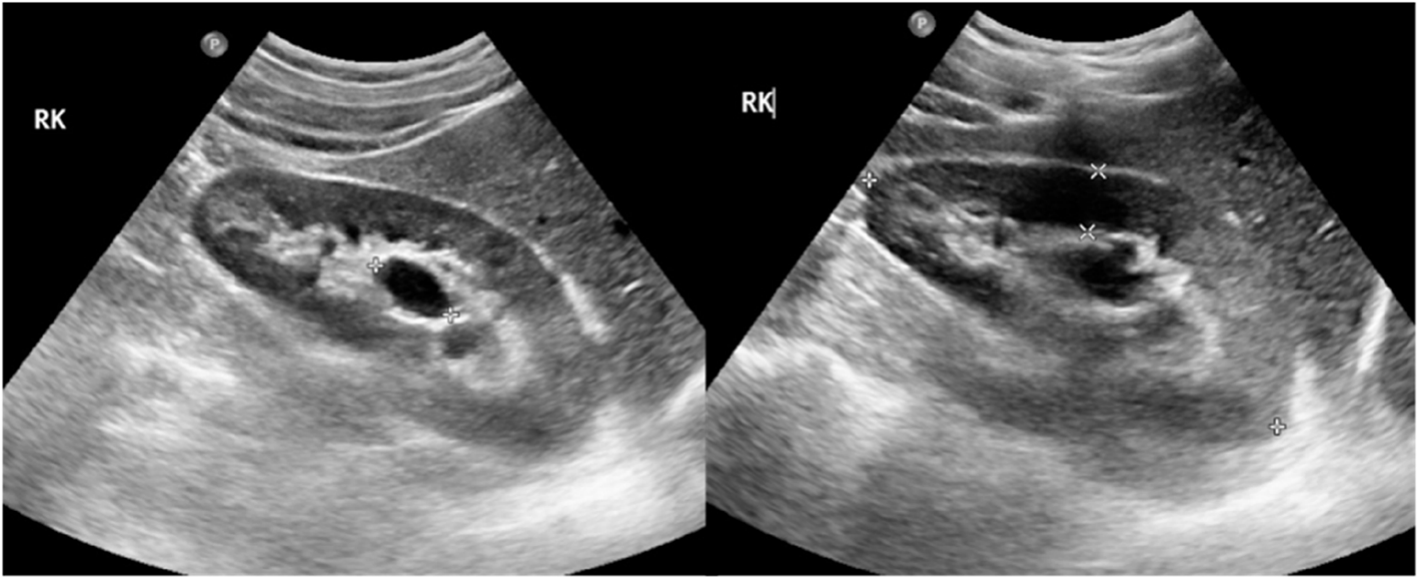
Ultrasound images of the right kidney showing cystic—like lesions
